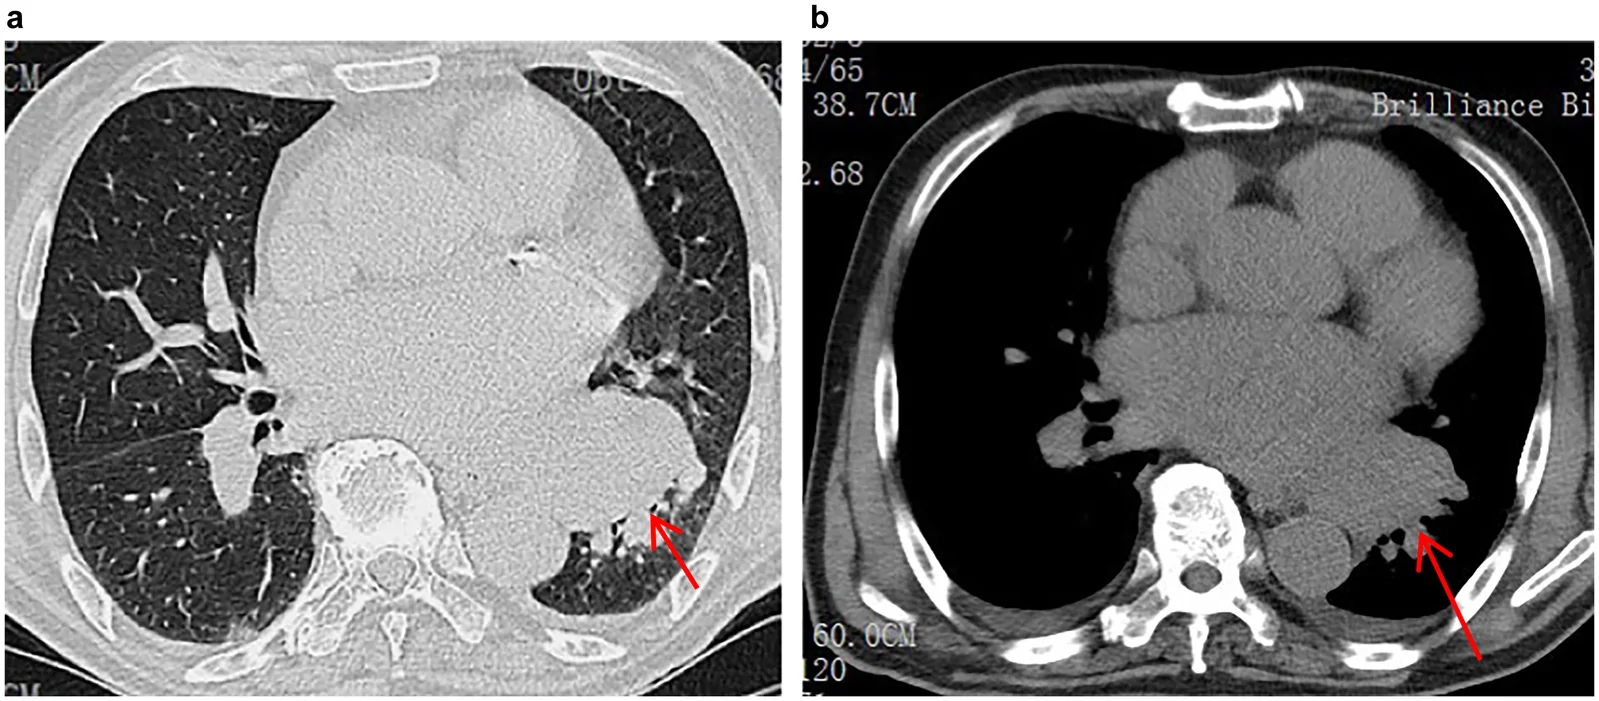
Plain chest computed tomography (CT) images. (a) Chest CT scan lung window. (b) Mediastinal window. A soft tissue mass was seen near the hilum of the lower lobe of the left lung. This mass showed unclear borders (red arrow), measured approximately 70 × 45 mm, and demonstrated a CT value of approximately 19–38 HU.
